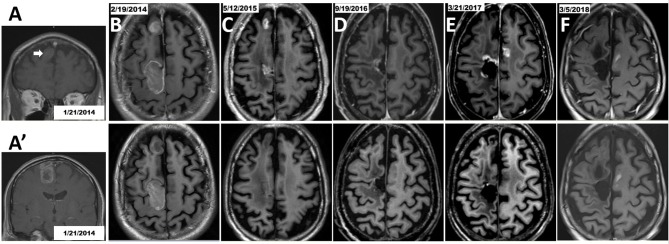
Therapy with TBI in combination with Gamma Knife stereotactic radiosurgery after the second GBM progression in patient number 5. Initial craniotomy was on 1/25/2014, but axial section images were not available in the pre-operative MRI. (A) Coronal view of anterior right frontal lesion (arrow). (A') Coronal view of posterior right frontal lesion. (B) Post-operative MRI. Tumor in the posterior right frontal lobe was removed while the mass at the right anterior frontal lobe was untouched. (C) MRI on 5/12/2015 showed that tumor in the right anterior frontal lobe progressed as a non-targeted lesion while on EF-14 clinical trial, and this lesion was treated with Gamma Knife on May 20, 2015. The patient had been treated with TBI therapy for 1 year and then it stopped as scheduled. (D) MRI on 9/19/2016 suggested tumor progression around the surgical cavity and he had a 2nd craniotomy on 9/25/2016. But pathology demonstrated no active tumor. This was not considered as progression of disease. (E) The 2nd disease progression was diagnosed based on MRI (3/21/2017) because of an appearance of a new lesion in the left hemisphere which was treated with Gamma Knife on 4/5/2017. The TBI regimen was restarted. (F) The progressive disease was well-controlled based on the latest MRI on 3/5/2018; lesions were treated with Gamma Knife followed by TBI regimen plus TTFields. Brain MRI T1 with contrast shown in upper row and brain MRI T1 without contrast shown in the corresponding lower row.
